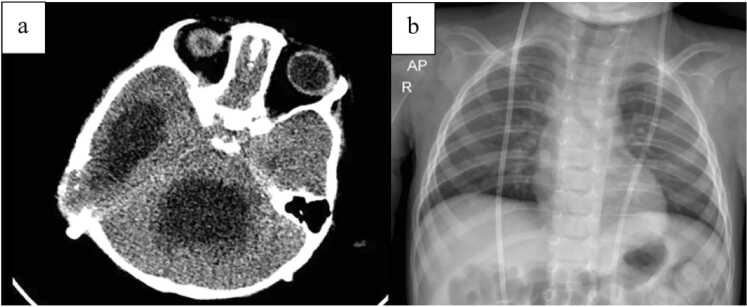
Cranial computed tomography and chest X-ray demonstrating the absence of pulmonary opacities or infiltrative lesions, with no radiologic evidence of pulmonary fungal involvement.
